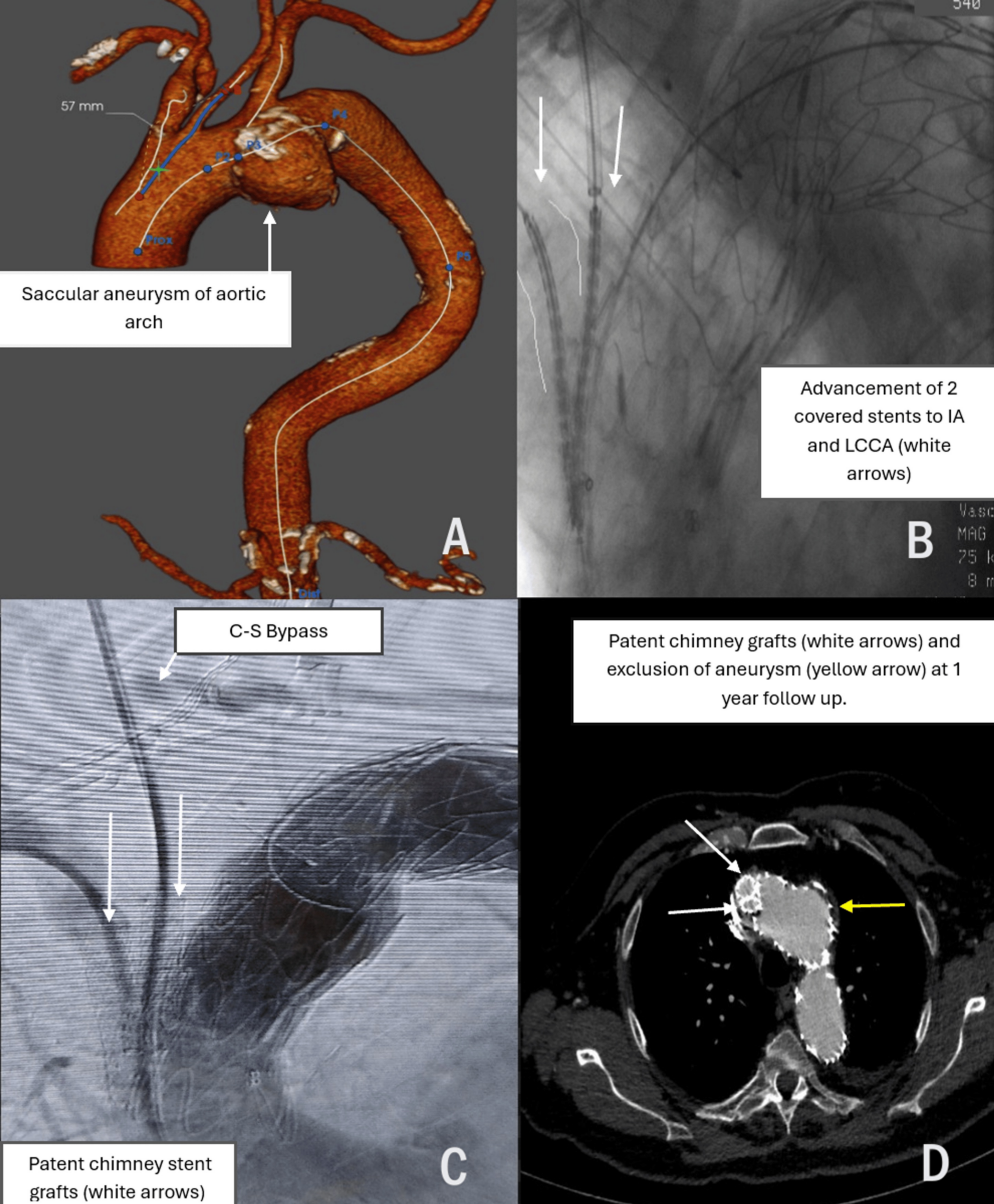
Saccular aneurysm of the aortic arch treated with double chimney stents and carotid-subclavian bypass. A. A male patient (77 years old) with a saccular aneurysm of the inner curve of aortic arch. B. Double chimney to innominate and left common carotid artery. C. Final angiography depicted exclusion of aneurysm with patent chimney stent grafts and left carotid-subclavian bypass (C-S bypass). D. Computed tomography angiography follow-up at one year showed exclusion of aneurysm and patent chimney-covered stents. LCCA: left common carotid artery.
